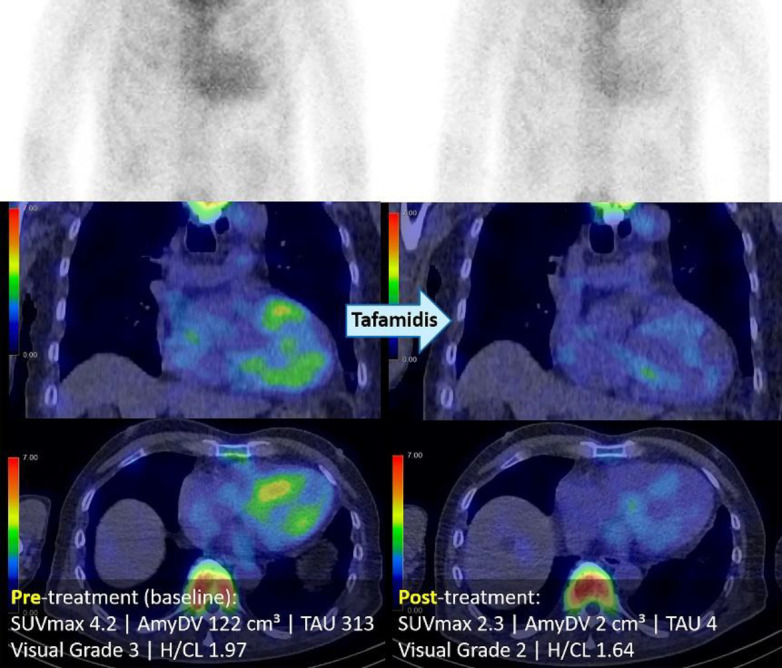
^99m^Tc-PYP planar and SPECT/CT images before (left) and after (right) tafamidis therapy. Marked visual and quantitative reductions in myocardial uptake are evident post-treatment. Quantitative indices (SUV_max_, AmyDV, TAU, and H/CL ratio) were derived using GI-BONE software (AZE Co., Ltd., Tokyo, Japan)
